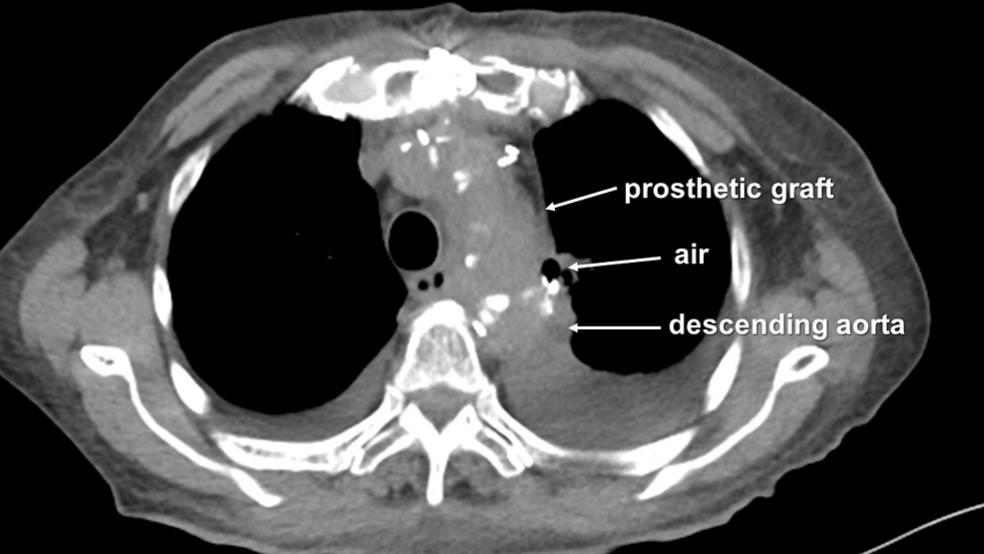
Second postoperative enhanced computed tomography scan revealing air around the prosthetic graft
